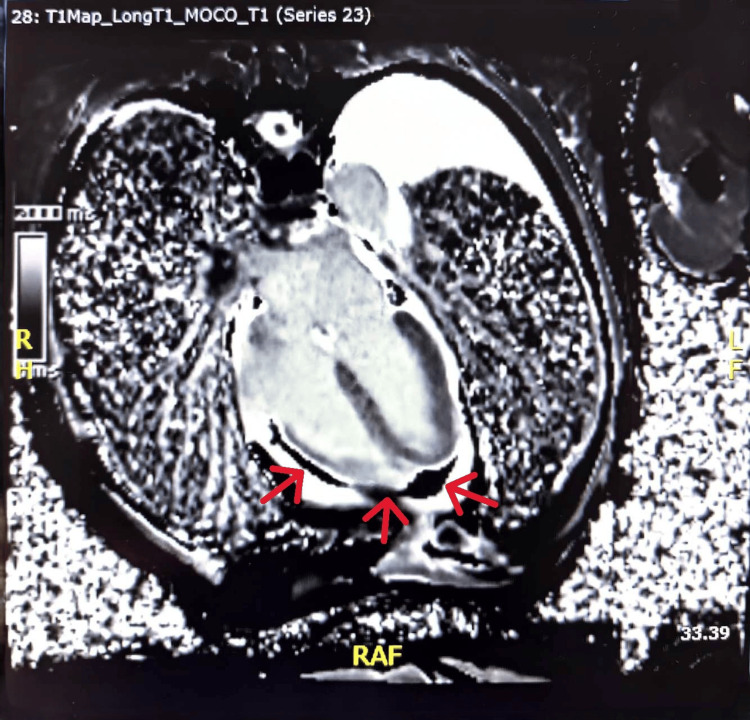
Cardiac MRI Demonstrating Pericardial Effusion and Inflammation Cardiac magnetic resonance imaging (MRI) revealed mild pericardial thickening (up to 5 mm) with late gadolinium enhancement (red arrows), indicating active pericardial inflammation. No myocardial edema or delayed myocardial enhancement was observed, and left ventricular systolic function was preserved (left ventricular ejection fraction (LVEF) 78%). A small left-sided pleural effusion was also noted. These findings supported inflammatory pericarditis without myocardial involvement and helped exclude constrictive physiology.
